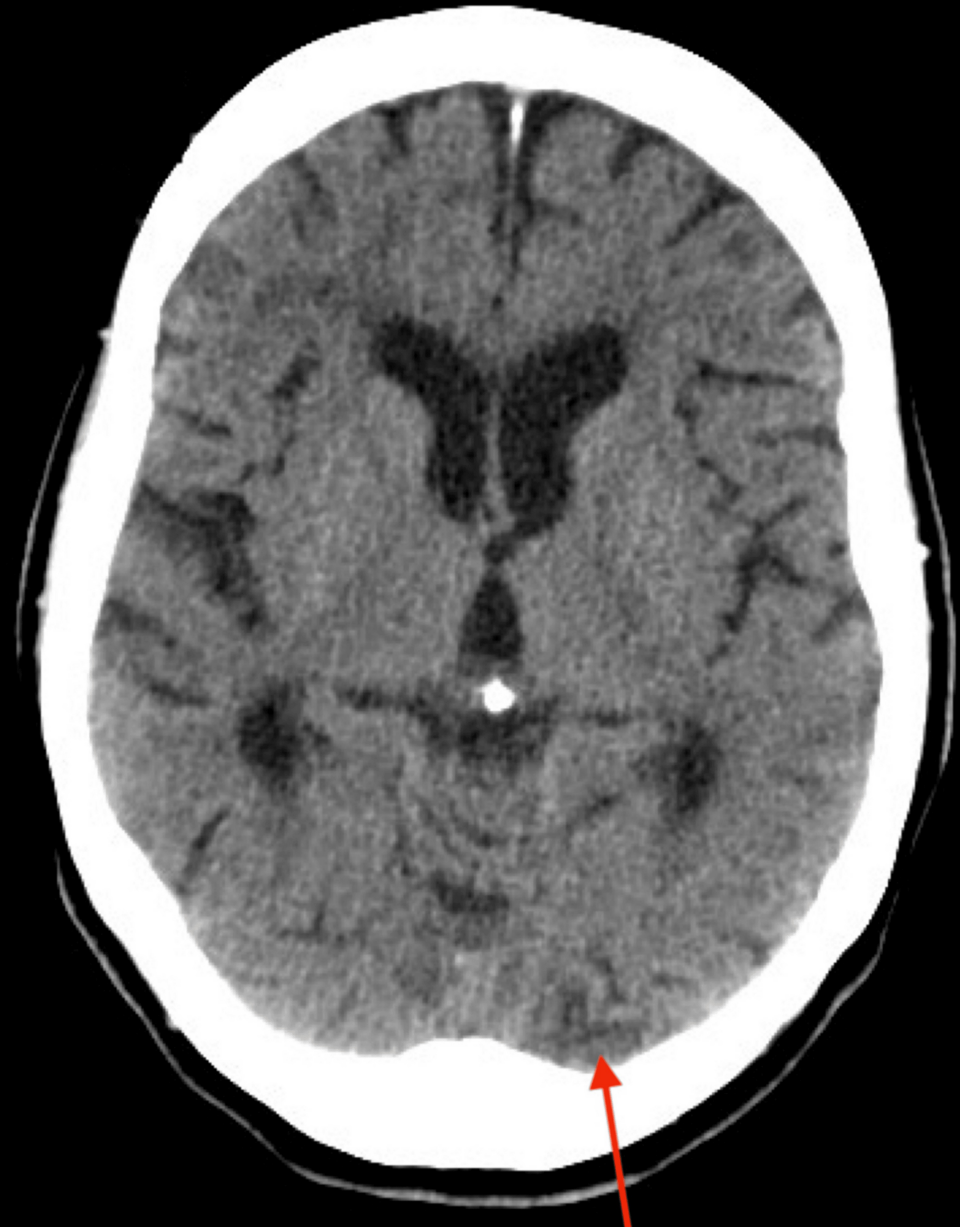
Left occipital lobe encephalomalacia Non-contrast axial CT scan of the brain demonstrating focal encephalomalacia in the left occipital lobe (red arrow), suggestive of a remote infarct.
